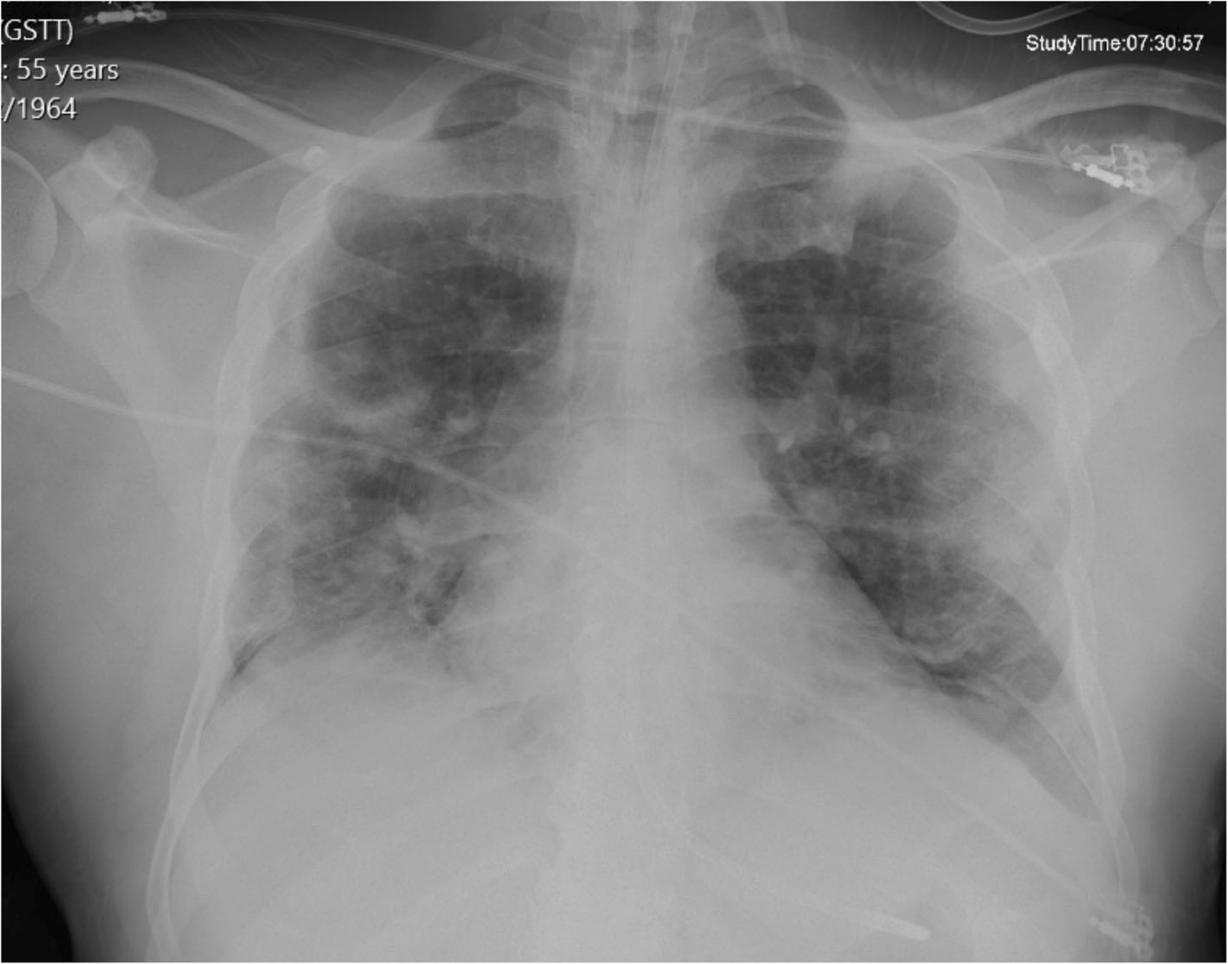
AP erect chest radiograph Legend - AP erect chest radiograph showing bilateral air space opacifications predominantly in the mid and lower zones
